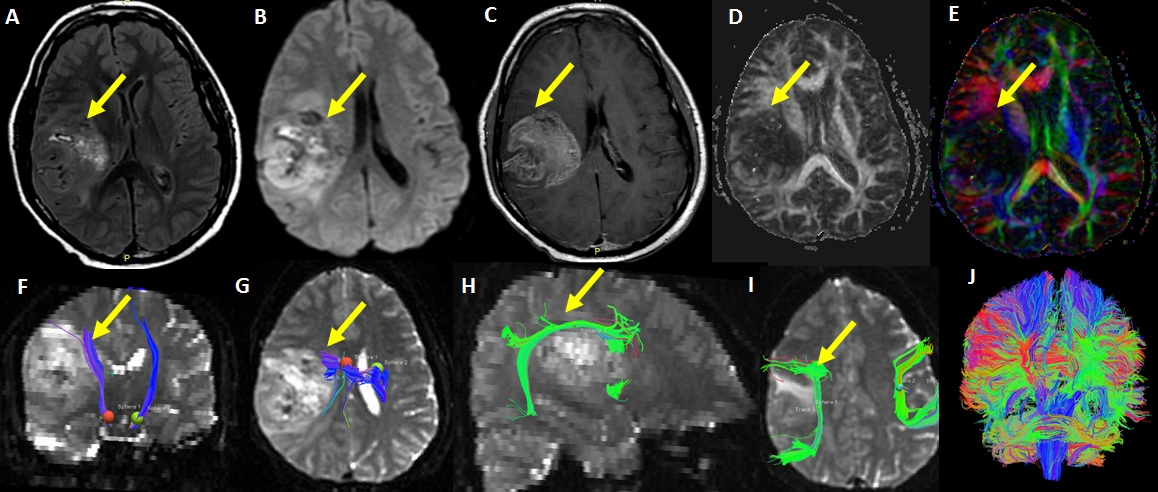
A 24-year-old male with right frontal-parietal glioblastoma FLAIR (A) DWI (B) Post contrast T1-W (C) images show a large enhancing tumor in the right frontal-parietal region. FA (D) and color-coded map (E) show decreased FA and destruction of white matter fibers. 3-D Tractography (F, G) was initiated from the brain stem (red and green sphere). The right corticospinal tract is shown passing through the anteromedial part of the lesion (arrow). The CST is also compressed and deviated anteromedially due to the lesion. 3D Tractography (H, I) of shows the arcuate fasciculus (arrow) compressed and deviated superiorly by the lesion and close to the tumor margin. Coronal whole brain tractography (J) of the same is shown.
